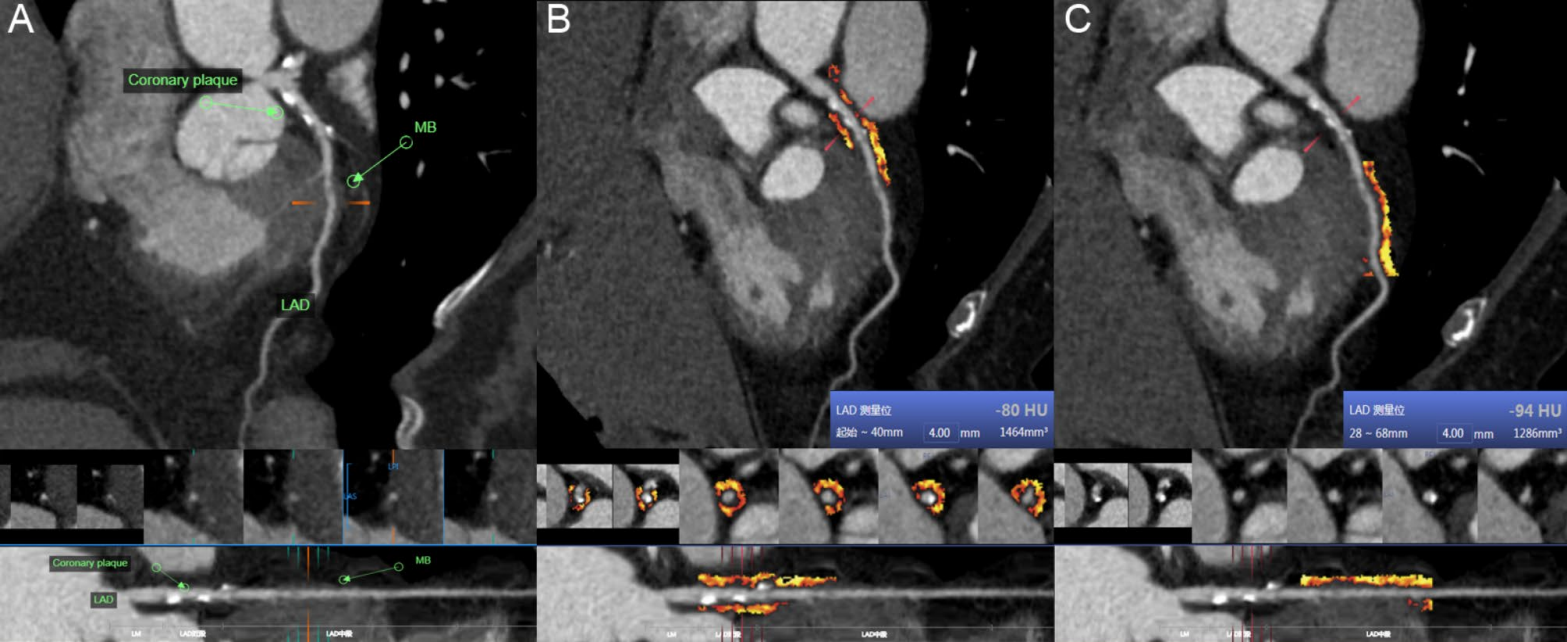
A 59-year-old male underwent CCTA. (a) The patient had an MB on the left anterior descending artery and coronary plaque in the segment proximal to the MB. (b) The FAI_lesion_ value for the 40-mm segment around the coronary plaque proximal to the MB was − 80 HU. (c) The FAI_MB_ value for the 40-mm segment around the MB was − 94 HU. *CCTA*: coronary computed tomography angiography, *MB*: myocardial bridge, *FAI*: fat attenuation index
